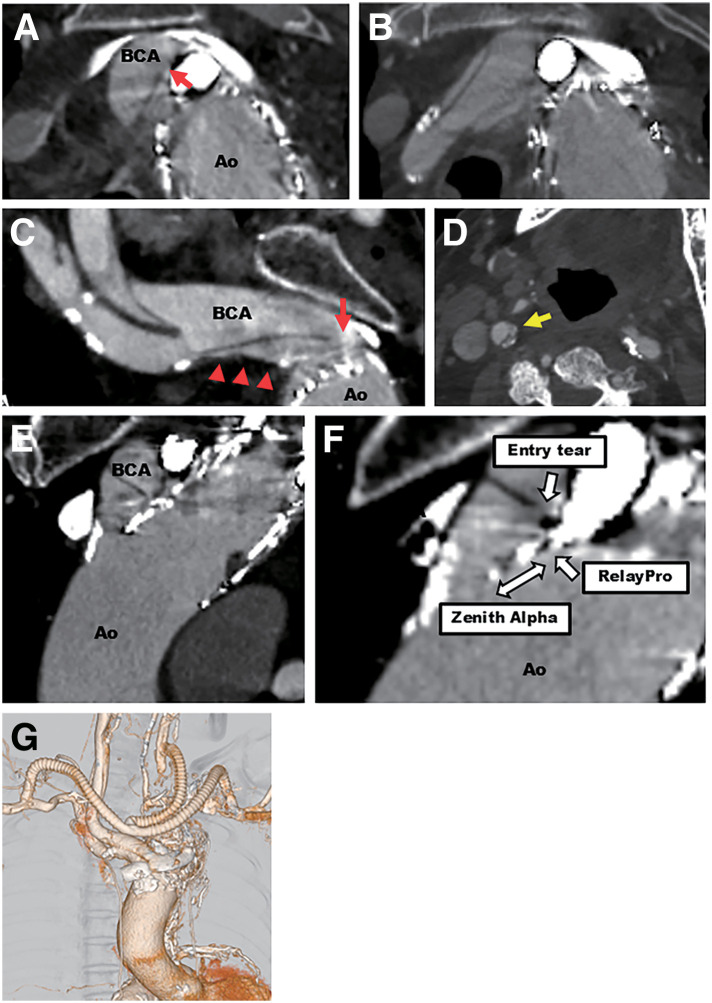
Contrast-enhanced computed tomography performed on postoperative day 5 at the onset of a transient loss of consciousness. (A, B) Axial and (C) coronal images showing dissection of the brachiocephalic artery with an entry tear near its origin (red arrow) and severe compression of the true lumen by the false lumen (red arrowheads). (D) Axial image at the level of the right internal carotid artery showing re-entry (yellow arrow) with preserved distal flow despite true lumen narrowing. (E) Oblique view confirming that the dissection is localized to the BCA. (F) Positional relationship between the stent grafts and the entry tear. (G) Volume-rendering image showing enhancement of the debranching bypass graft. Ao: aorta; BCA: brachiocephalic artery
